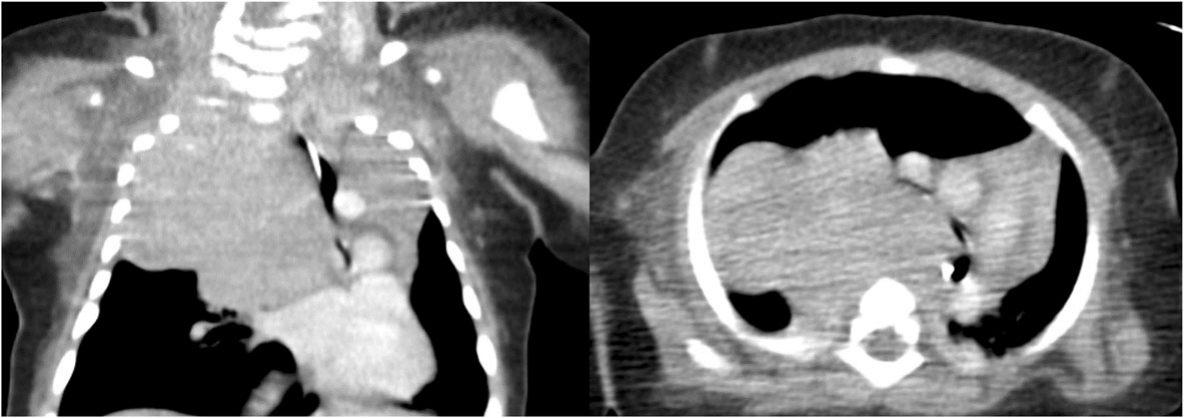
Computed tomography images of a patient undergoing subtotal tumor resection with extracorporeal membrane oxygenation stand-by. The huge posterior mediastinal tumor (neuroblastoma) is compressing the trachea and right main bronchus
